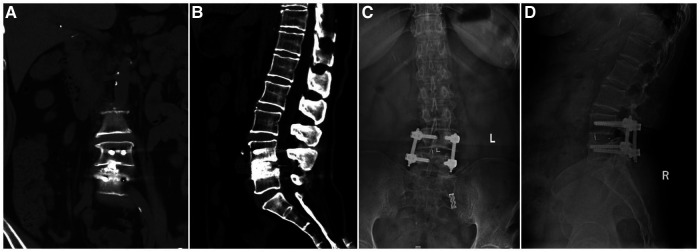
Imaging findings during follow-up of a patient who underwent UBE-TLIF. (A,B) Coronal and sagittal CT showing that the cage was well positioned and high-density bone fusion between vertebral bodies 6 months after the operation. (C,D) 13-month postoperative x-ray showing bony fusion and that the instrumentation was in a good position.
